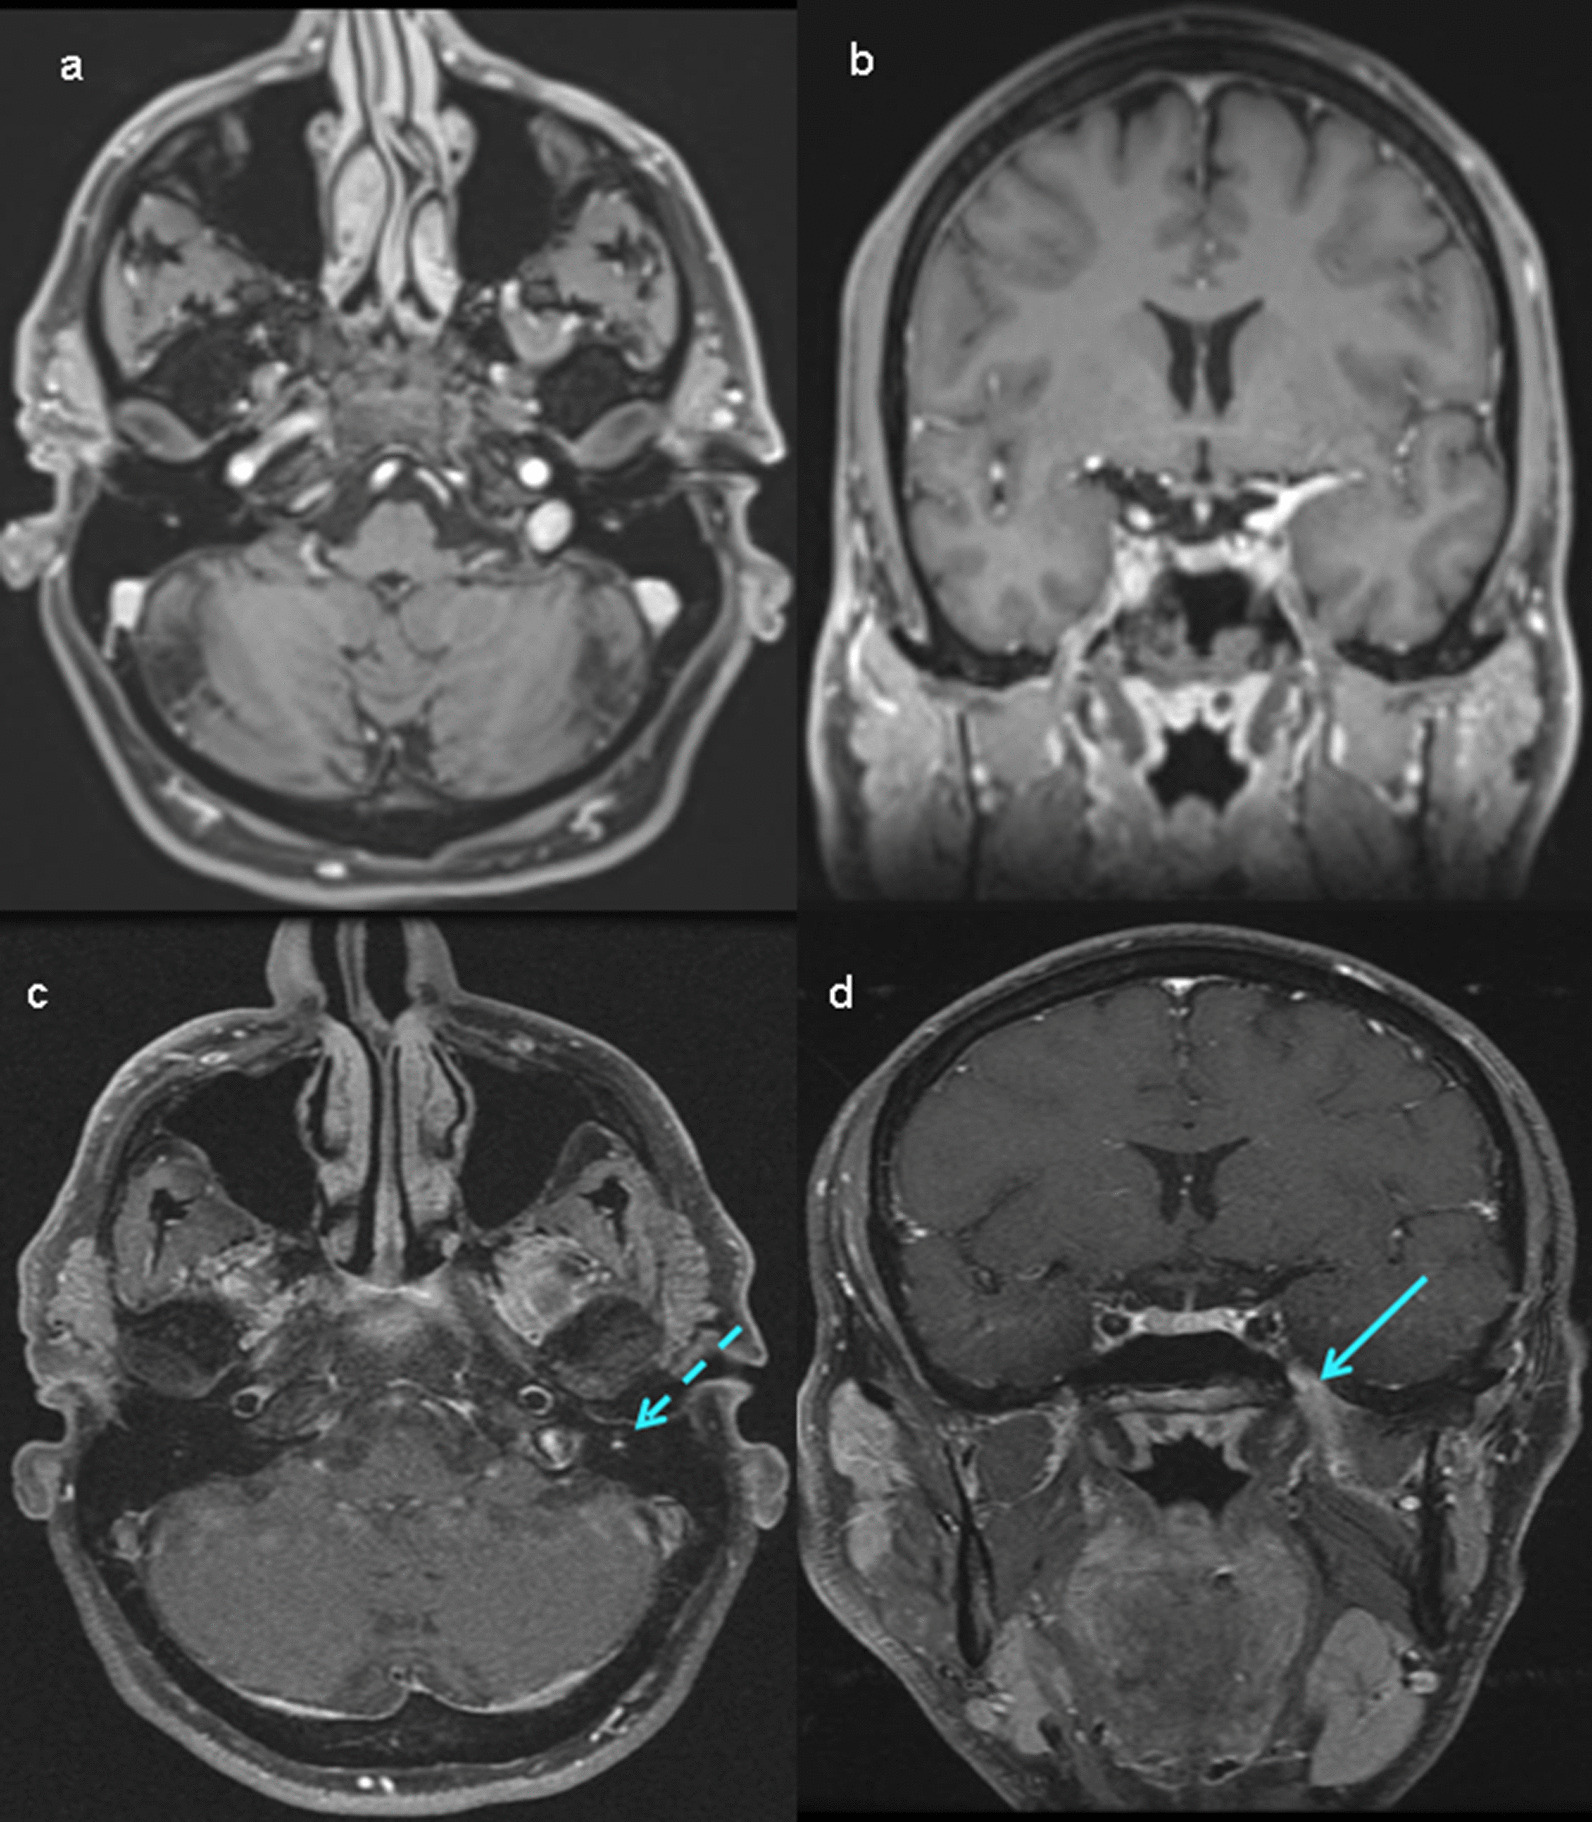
Comparison between initial and follow-T1 Post-gadolinium MR imaging in patient 3 a axial view b coronal view. No abnormality identified. MRI 6 months later c axial image shows abnormal asymmetric enhancement in the mastoid segment of left facial nerve (dashed arrow) d coronal image shows extension along the V3 branch of the left trigeminal nerve (arrow)
